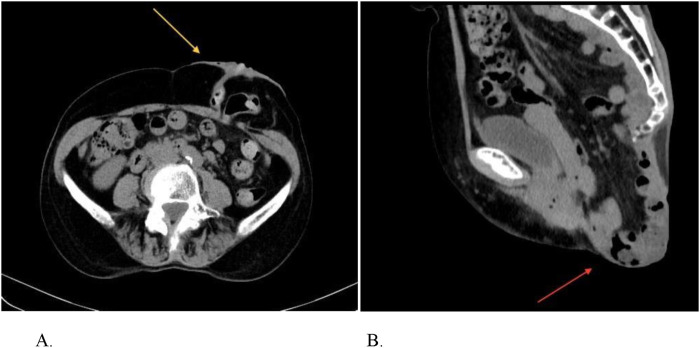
Pre-operative CT abdomen/pelvis showing the hernia defects. (A) Parastomal hernia. (B) Perineal hernia.
